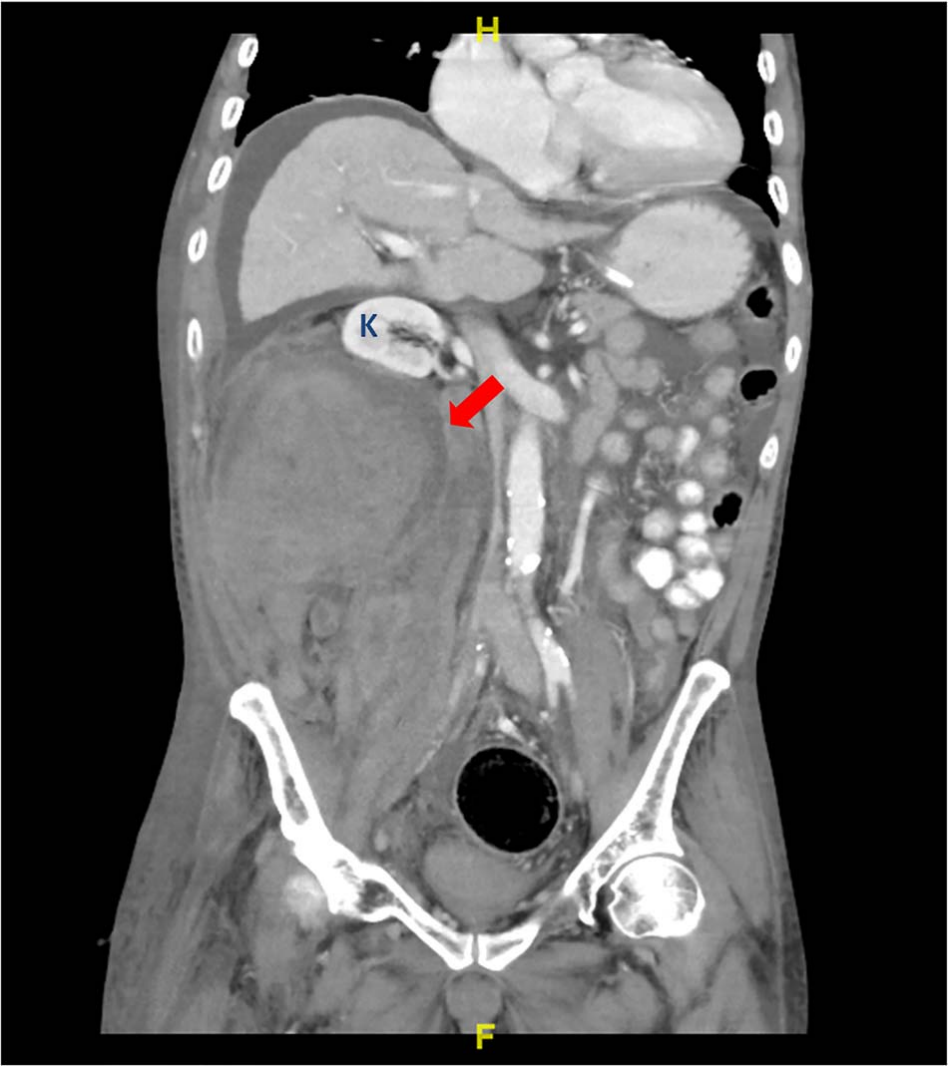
CT abdomen coronal view shows large right retroperitoneal hematoma (red arrow) involving right psoas muscle displaced right kidney (K) anterosuperiorly.
